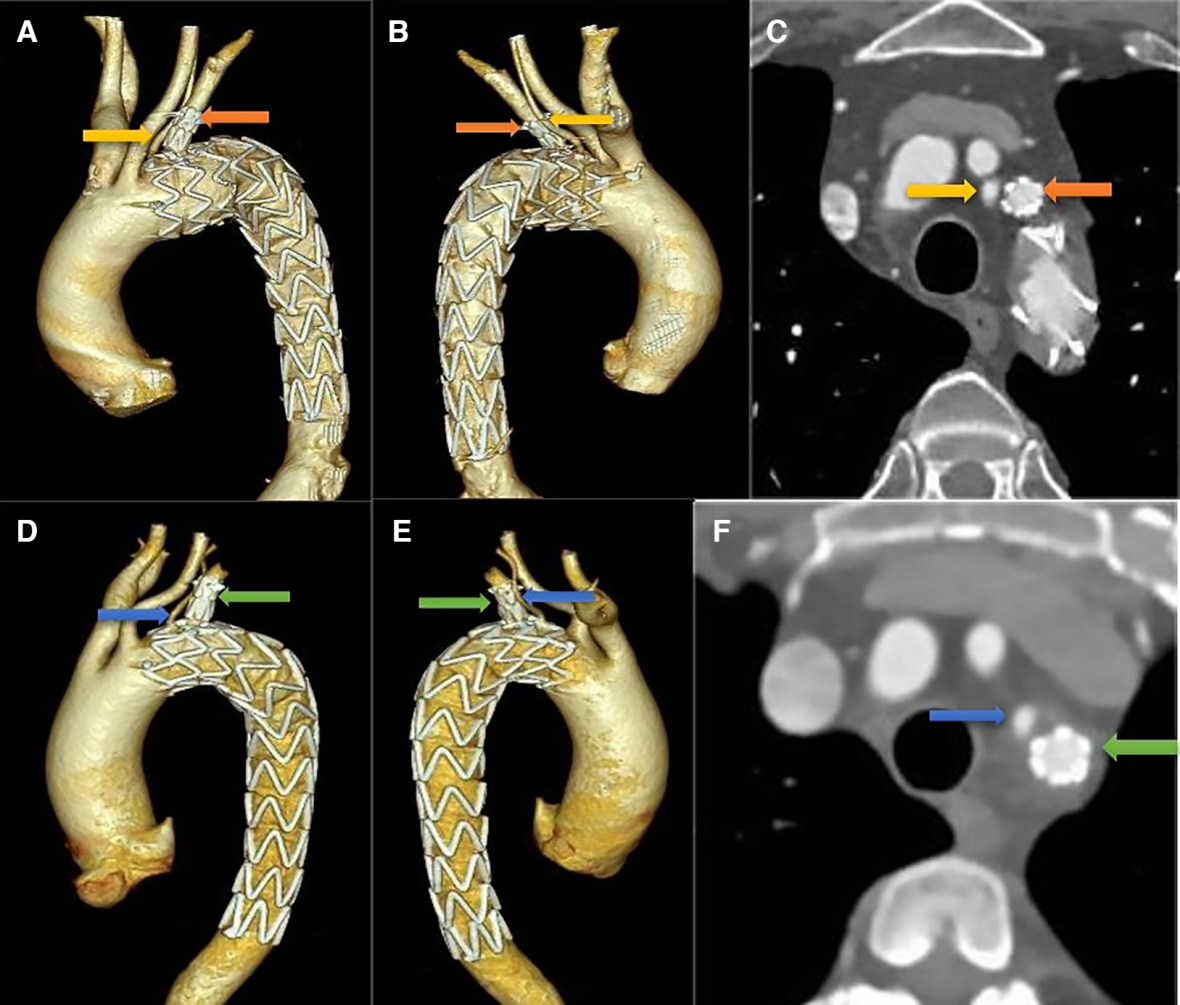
Follow-up CTA imaging. (A–C) in a patient with thoracic aortic aneurysm, both ILVA and LSA maintained patency without stenosis on three-dimensional reconstruction and cross-sectional images; (D–F) the same results can be seen in a patient with type B intramural hematoma.
